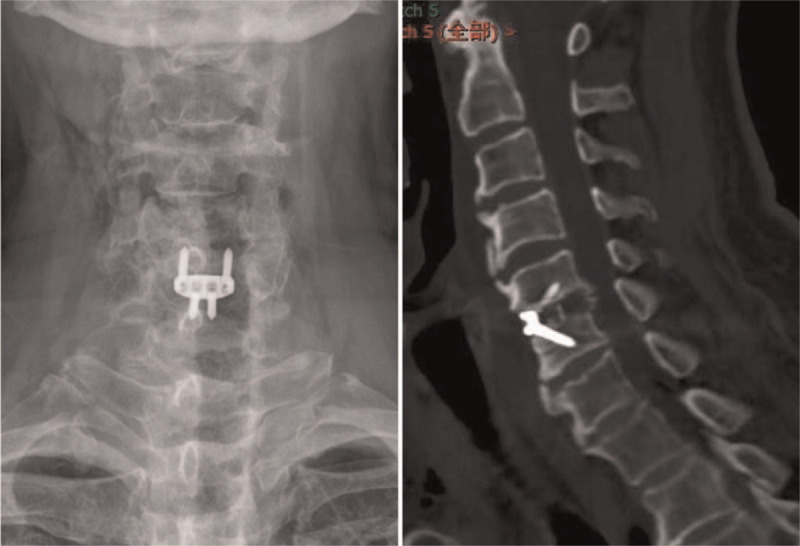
Postoperative imaging of the cervical spine. X-ray (A) and CT (B) showed most of the osteophytes in C4/5 and C6/7 were removed and the fusion cage was in good position. CT = coronal and sagittal computerized tomography.
